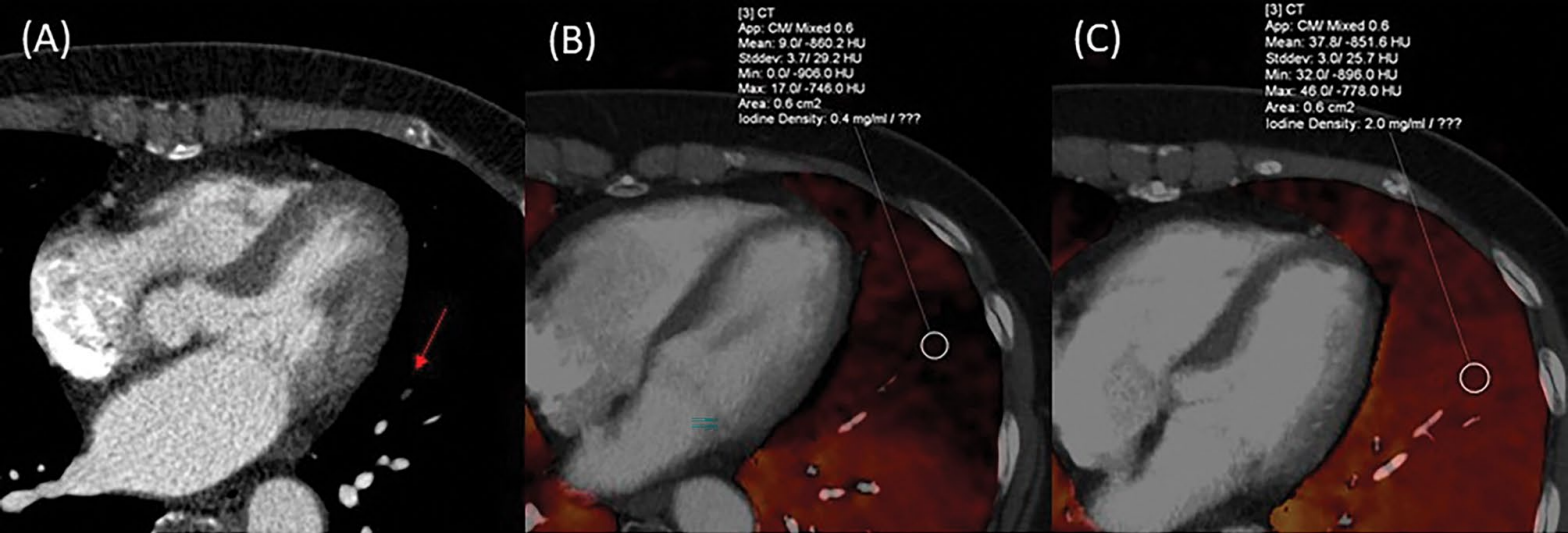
A 51-year-old-man with pulmonary embolism. (A) Peripheral occlusive thrombi can be seen in left A4a on the pre-treatment computed tomography pulmonary angiography (CTPA). (B) Pre-treatment perfused blood volume shows perfusion decrease in the left S4a. The mean HU value of the segment is 9.0 HUs. (C) Lung perfusion blood volume improved after treatment. The mean computed tomography value of the segment is 37.8 HUs.
